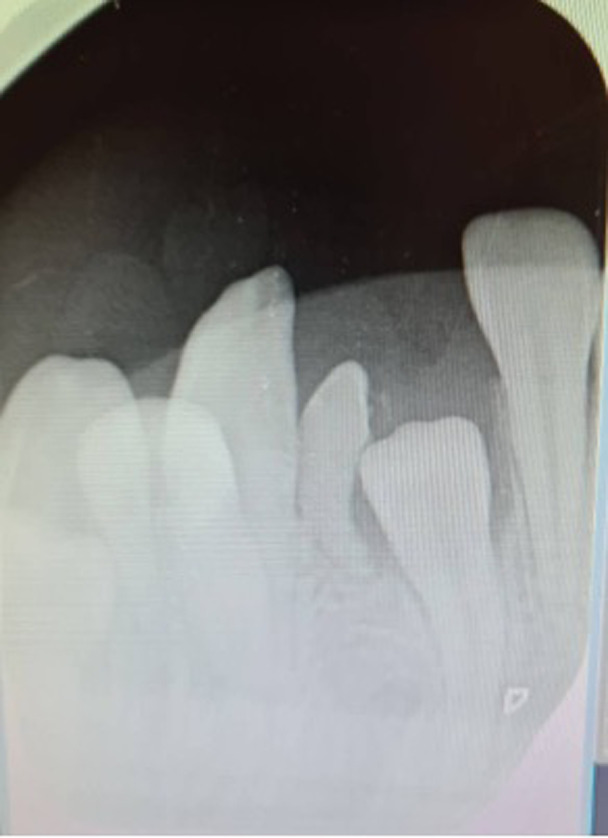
X-ray control, one last odontoma at the bottom
